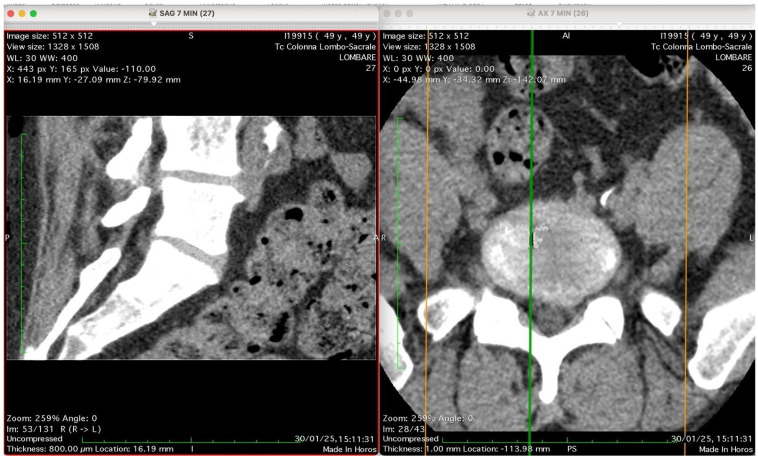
Computed tomography (CT) images of the lumbosacral spine at 0 min post-injection, shown in sagittal (left) and axial (right) planes. No visible gas is present in the intervertebral discs at this early time point. The sagittal view shows vertebral bodies, discs, and spinal canal; the axial slice confirms anatomical alignment. These baseline images serve as reference for gas migration tracking following paravertebral oxygen–ozone injection. Technical details: 512 × 512 matrix, 0.8 mm slice thickness, WL/W: 30/400, scan date: 30 January 2025.
